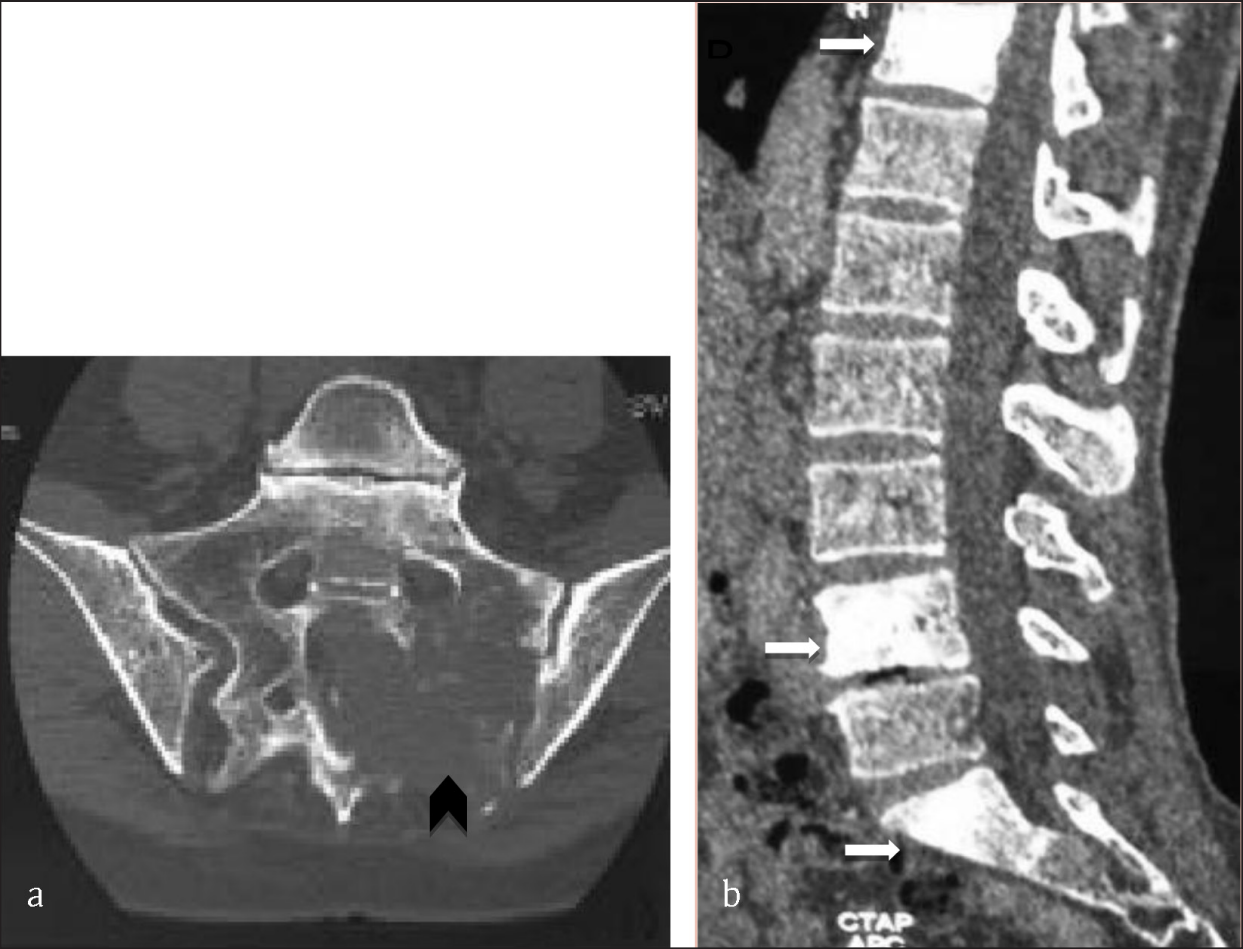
Lymphoma of the sacrum. Axial CT scan image shows a destructive lesion of sacrum extending through the left sacroiliac joint (arrowhead).
